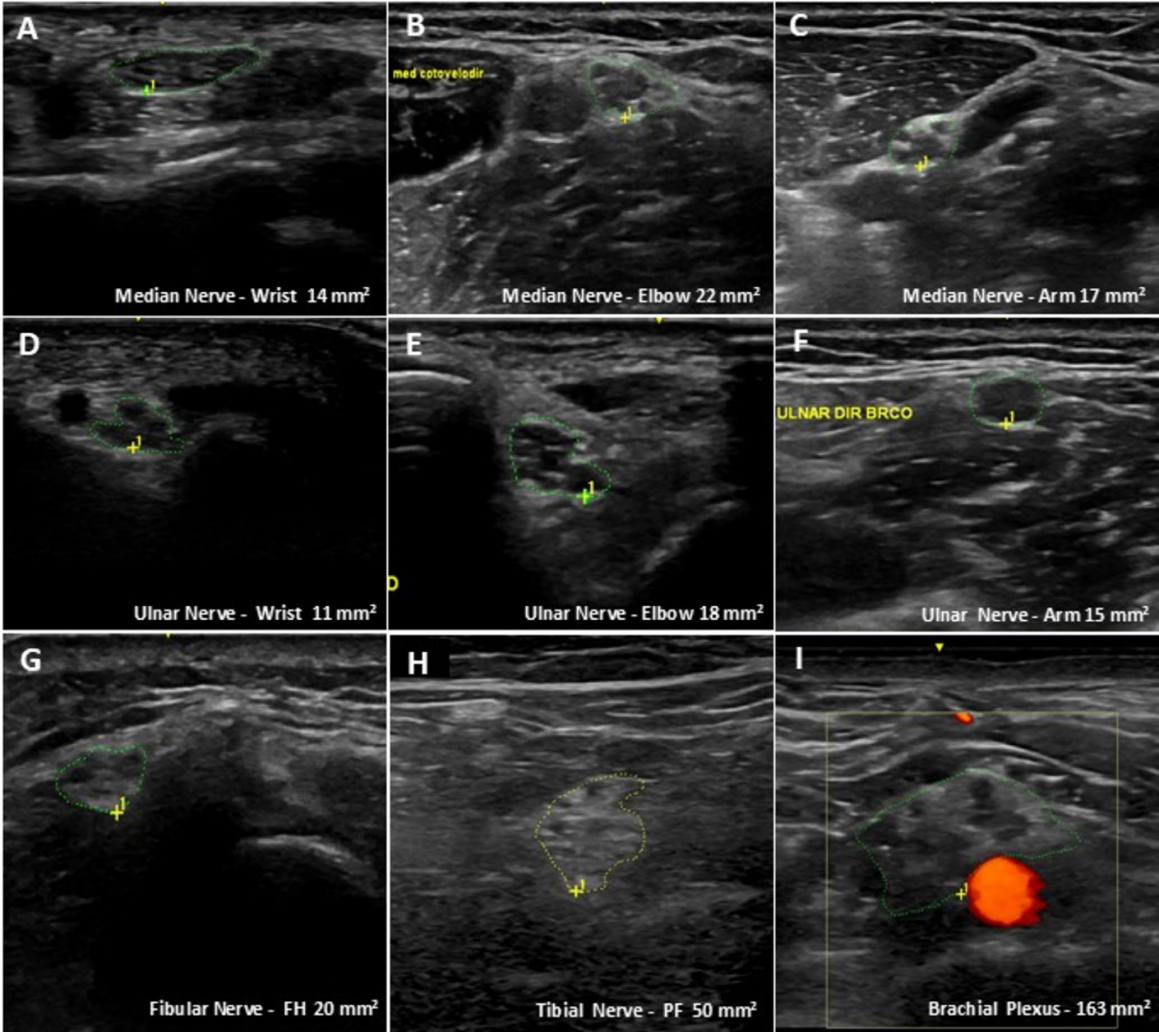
Nerve ultrasound findings in a patient with cerebrotendinous xanthomatosis. Representative ultrasound images demonstrating peripheral nerve enlargement in CTX—Patient 6. Color outlines (yellow and green) indicate the nerve boundaries used for cross‐sectional area (CSA) measurements (in mm^2^). Panels show: (A) Median nerve at the wrist (14 mm^2^), (B) Median nerve at the elbow (22 mm^2^), (C) Median nerve at the arm (17 mm^2^), (D) Ulnar nerve at the wrist (11 mm^2^), (E) Ulnar nerve at the elbow (18 mm^2^), (F) Ulnar nerve at the arm (15 mm^2^), (G) Fibular nerve at the fibular head (20 mm^2^), (H) Tibial nerve at the popliteal fossa (50 mm^2^), and (I) Brachial plexus at the supraclavicular level (163 mm^2^).
